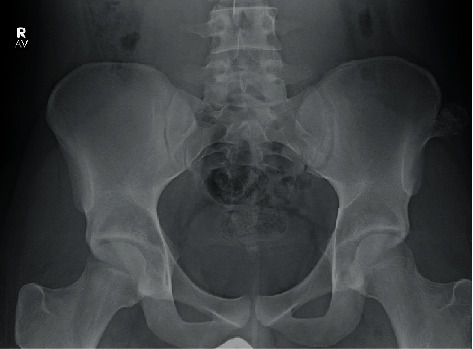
Preoperative anterosuperior pelvis plain-film radiograph. Radiograph demonstrates an exophytic mass growing laterally from the left iliac wing measuring 2.8 × 3.1 cm (Osteochondroma pre-op.jpg).
